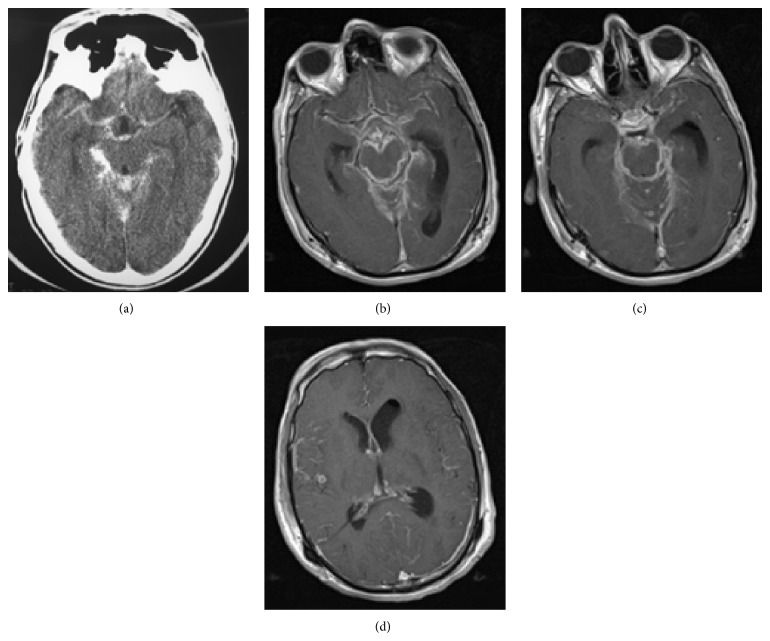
Meningeal tuberculosis. (a) Axial postcontrast brain CT shows typical thick enhancement of basilar cisterns. (b) Axial postcontrast T1-weighted MR images in a different patient ((b), (c), and (d)) demonstrate enhancing basilar exudates and leptomeningeal enhancement. A small tuberculoma in right temporal region (d) and hydrocephaly (more severe in left ventricle) and the evidence of prior craniotomy (Burr hole, left pneumoventricle) are also evident.
